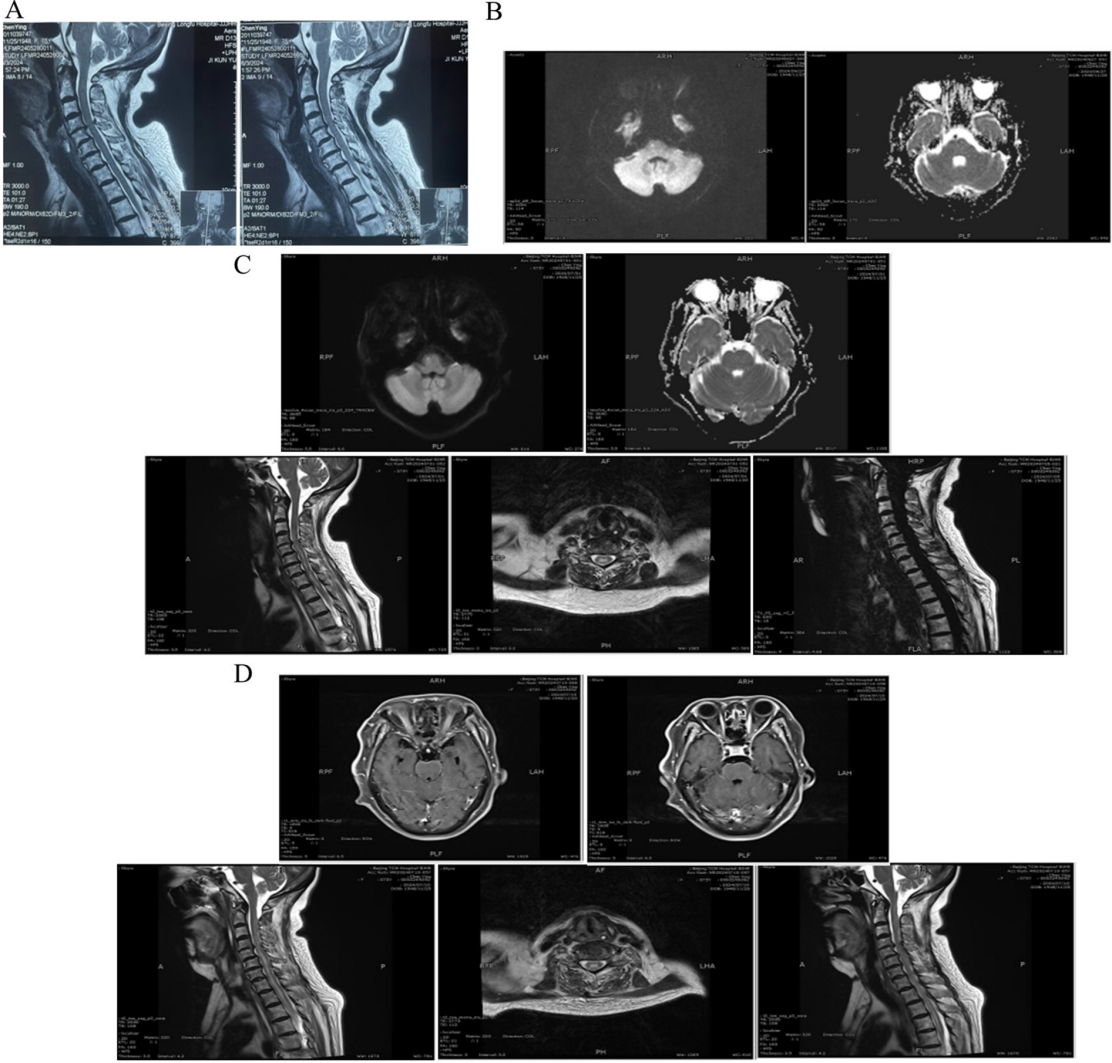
Cervical spine and brain MRI. (A) Preoperative cervical spine MRI: C3–7 spinal canal stenosis, C4–7 bilateral foraminal stenosis. (B) 27/6/2024 Brain MRI DWI and ADC image: the pons shows a minor, punctate, elevated signal. (C) 1/7/2024 Brain MRI DWI and ADC image: The cerebral bridge showed a small, punctate, elevated signal with no apparent diffusion coefficient (ADC) value change. 1/7/2024 Cervical spine MRI: The cervicothoracic medullary center at the C5–T2 level is distinguished by a striated high signal shadow on T2–weighted imaging. The dural sac at C4–C7 is compressed, the spinal canal is constricted at the horizontal level, the cervical medulla is compressed, and the paravertebral soft tissues show no abnormal signals. (D) 10/7/2024 Brain MRI T1WI: The meninges of the pontine and periaqueductal regions exhibit mild thickening and significant enhancement. 10/7/2024 Cervical spine MRI: No anomalous signals were detected in the cones; however, there is evidence of dural sac compression at C4–C7, spinal canal narrowing at that level, cervical medulla compression, and no abnormal signals in the paravertebral soft tissues. A prominent hyperintense shadow was noted on T2–weighted images throughout the cervical medulla and at the center of the thoracic medulla at the T1–5 level. The spinal cord was observed to be mildly thickened at the C4–T5 level.
